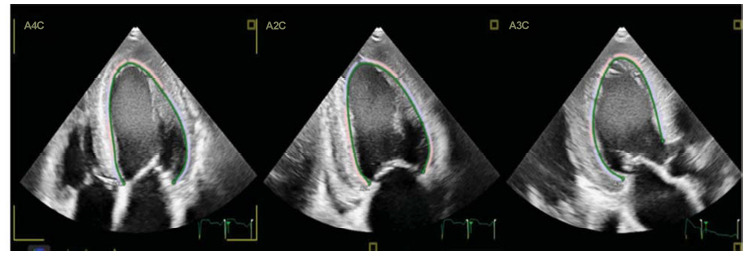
Left ventricular spontaneous echo contrast (LV-SEC) in a patient with severe LV systolic dysfunction. Smoke-like density appears on echocardiography in apical four (A4C), two (A2C), and three (A3C) chamber views, indicating stasis of blood in the left ventricle with low left ventricular strain.
